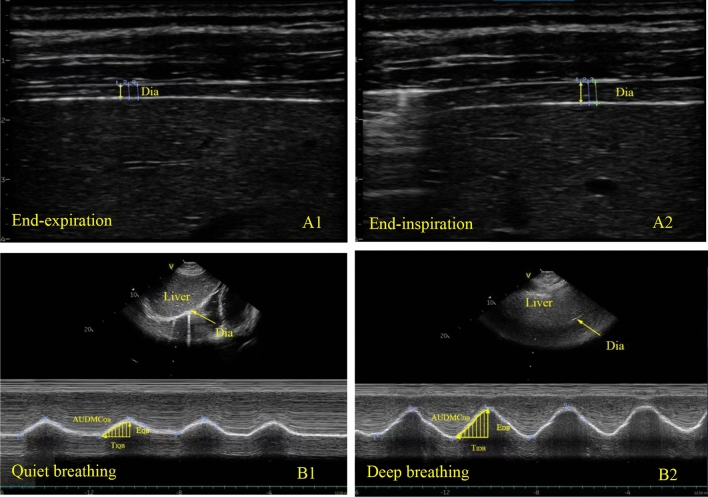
Diaphragmatic thickness measured by B mode ultrasound at end-expiration (A1) and end-maximum inspiration (A2). Diaphragmatic mobile curve in M mode of ultrasound was shown during quiet breathing (B1) and deep breathing (B2). Parameters were measured at three consecutive breathing cycles. EQB, excursion during quiet breathing; EDB, excursion during deep breathing; TI, inspiratory time; AUDMC, area under diaphragmatic movement curve in the inspiratory phase. Contractile velocity: diaphragmatic excursion/inspiratory time.
